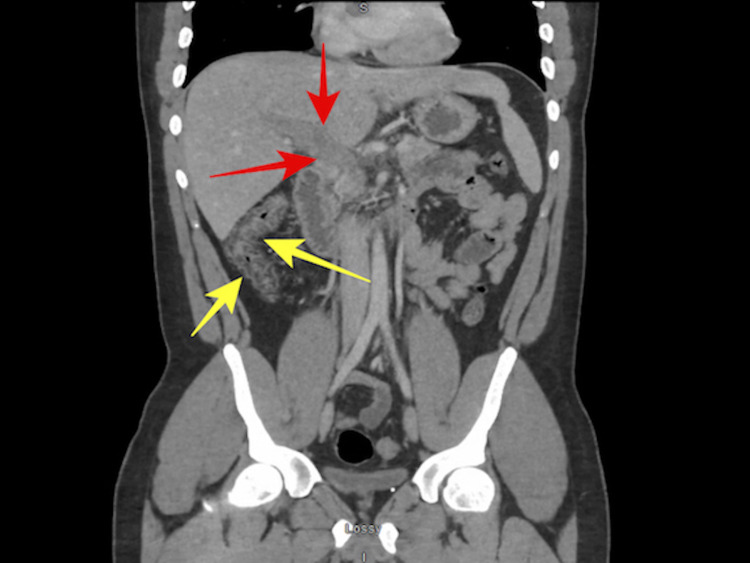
Coronal CT scan of the abdomen and pelvis shows an area of low attenuation in the region of portal vein suggestive of portal vein thrombosis (red arrows) and submucosal fat attenuation and signs of inflammation in the visualized portion of the colon suggestive of burned-out colitis or inflammatory bowel disease (yellow arrow).
